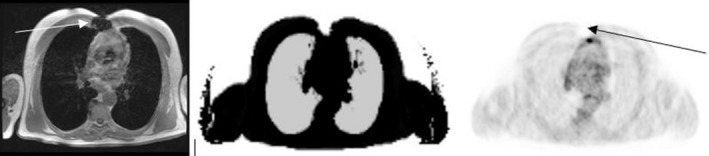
A susceptibility artifact evident in the chest wall on magnetic resonance imaging (Left, white arrow), the attenuation map (Middle), and the positron emission tomography (PET) image (Right). The lung appears continuous with the demarcated air pocket in the reconstructed PET image (black arrow).
